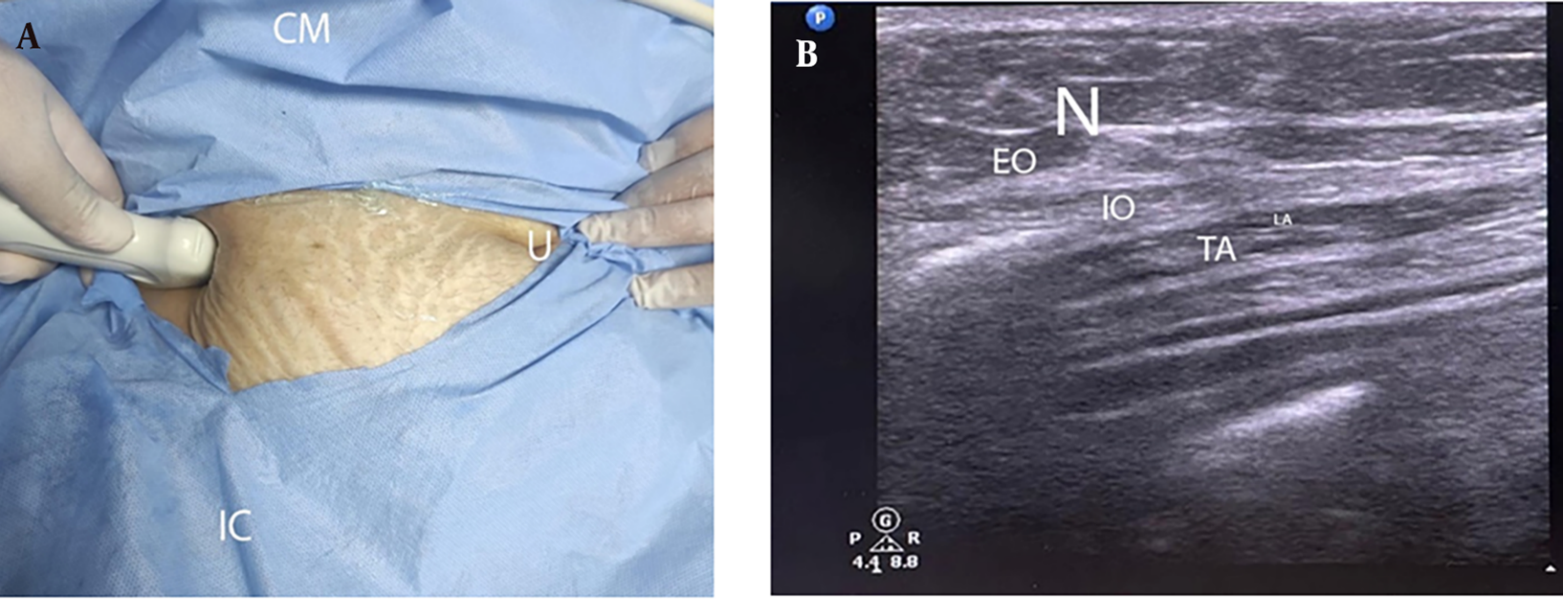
Transversus abdominis plane block technique (A) position and (B) ultrasound image (CM, costal margin; IC, iliac crest; U, umbilicus; N, needle; EO, external oblique muscle; IO, internal oblique muscle; TA, transversus abdominis muscle; LA, local anesthetic).
